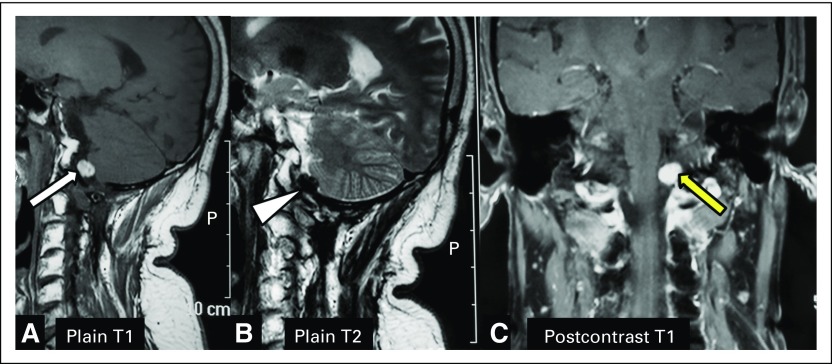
Follow-up images obtained after the second surgery (2014). Magnetic resonance imaging showed well-defined intradural extramedullary lesion at the cervicomedullary junction. On the plain T1-weighted sagittal view (A), the mass was hyperintense (white arrow); on the plain T2-weighted sagittal view (B), it showed hypo-intense signal intensity (arrowhead). The postcontrast T1-weighted coronal view (C) showed intense postcontrast enhancement (yellow arrow). The imaging findings described were diagnostic for melanoma and represented residual disease after the second surgery.
